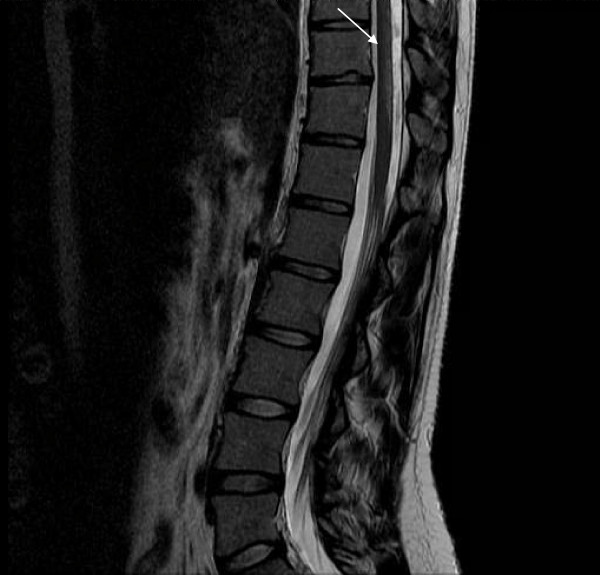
Sagittal T2-weighted MRI scan showing an area of hyperintensity involving the bilateral posterior and lateral columns of the thoracic and lumbar junction before treatment.
